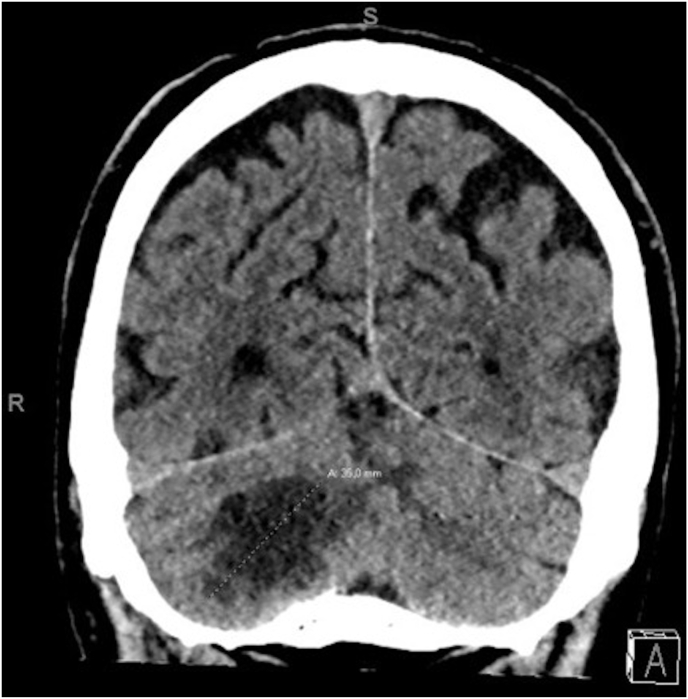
Coronal view of brain CT imaging demonstrating a solitary, partially necrotic lesion in the right cerebellar hemisphere. The maximum diameter of the cerebellar lesion measured 35.0 mm.
